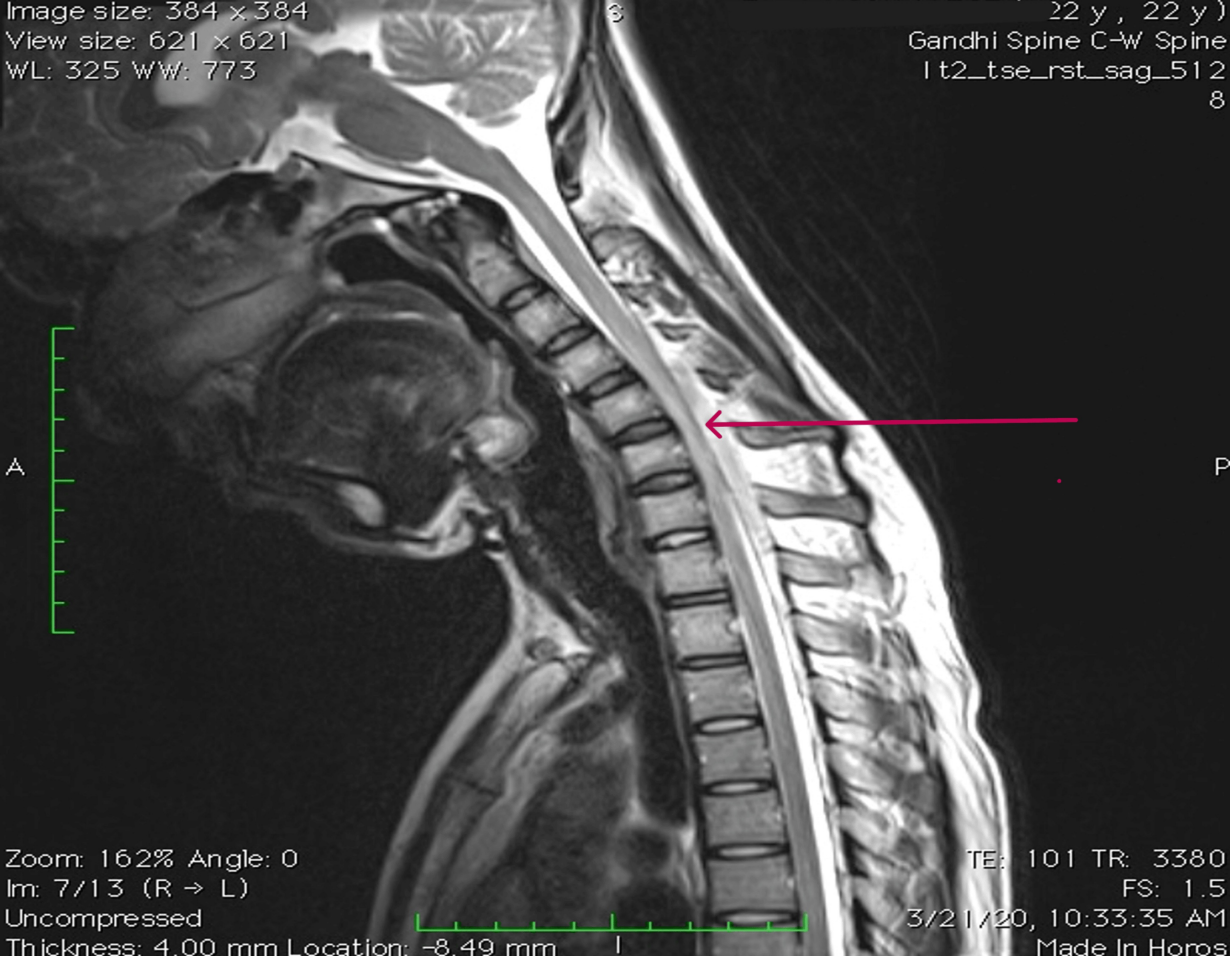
Abnormal T2W sagittal flexion MRI of the neck showing the loss of dural attachment and anterior displacement of the dorsal horn
